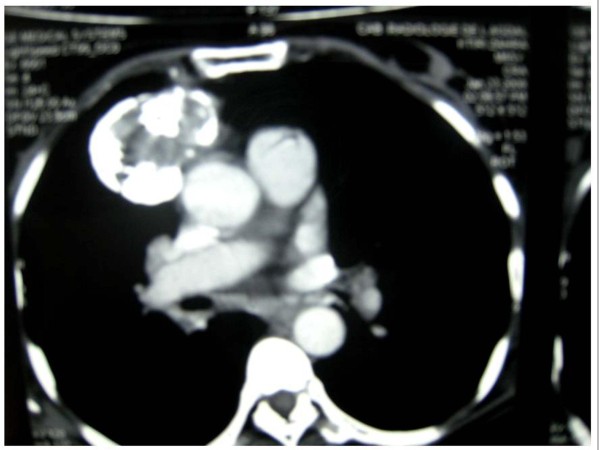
CT of thorax. It shows a round cystic tumor, with significant calcification in the right anterior mediastinum.
